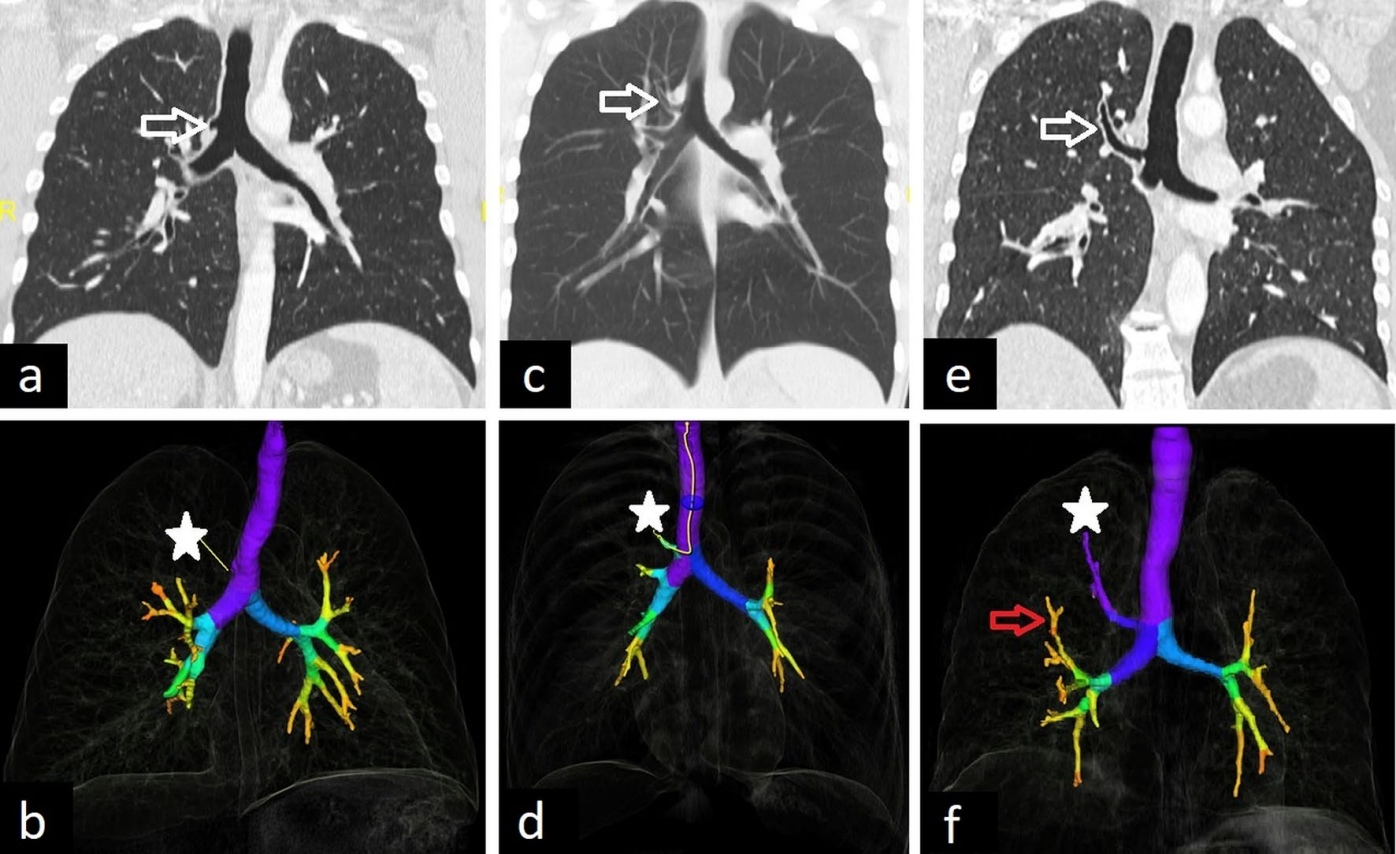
CT images of tracheal bronchi in different patients. Blind-ended tracheal bronchus is seen on coronal (a, arrow) and 3D reconstruction (b, star) CT images. Tracheal bronchi with accompanying lung tissue are demonstrated on coronal (c, e, arrow) and 3D reconstruction (d, f, star) CT images in two patients. The tracheal bronchus supplies the apical and posterior segments of the right upper lobe (e, arrow) and the right middle bronchus supplies the anterior segment of the right upper lobe (f, red arrow) in a 43-year-old asymptomatic male patient
